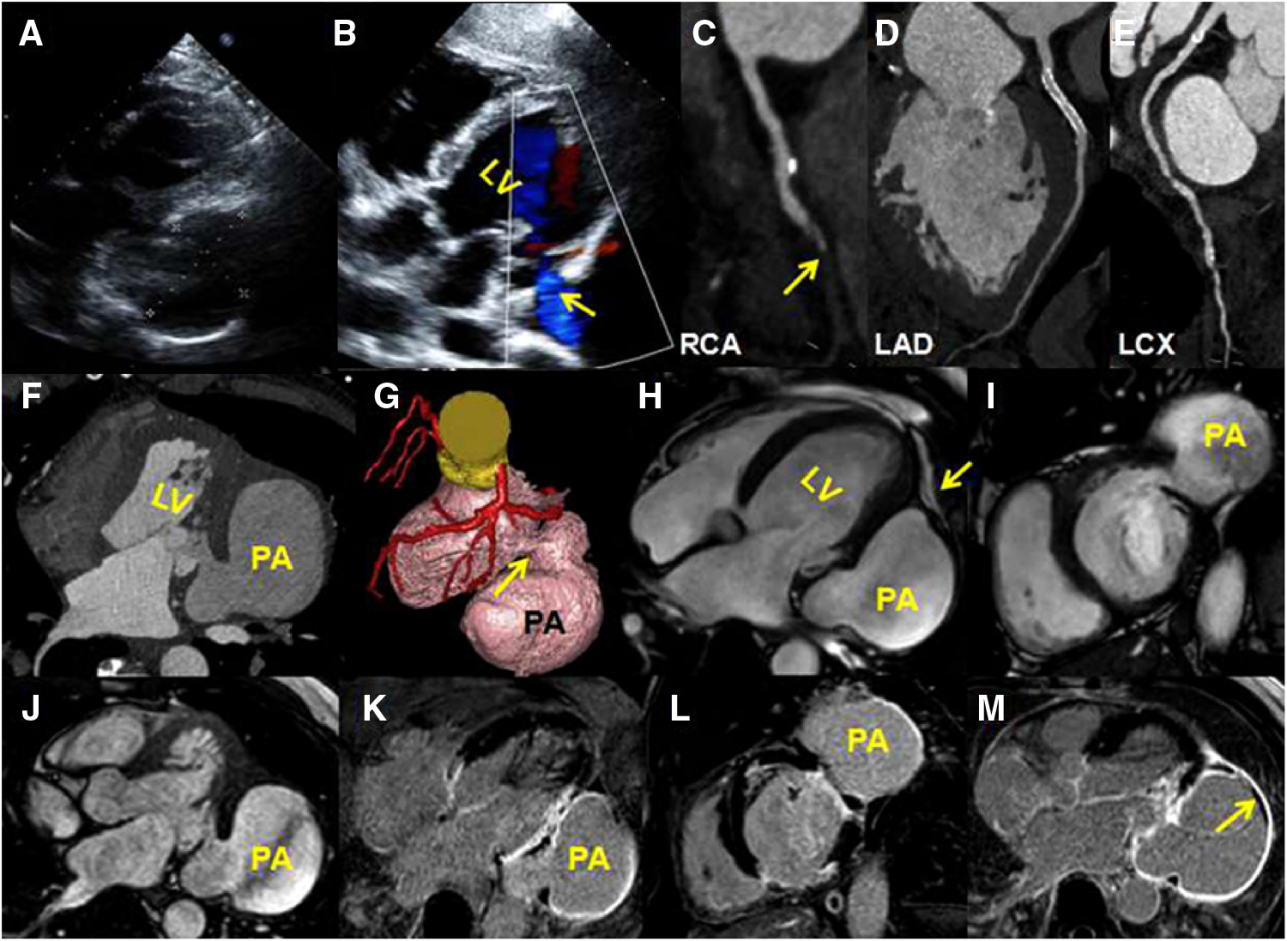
(A) A transthoracic four-chamber echocardiography image shows an aneurysm protruding from the posterior wall of the LV; the size of the aneurysm is 6.85 cm × 4.82 cm. (B) A color Doppler image shows a shunt from the left ventricle to the aneurysm (yellow arrow). (C–E) A coronary computed tomography angiography of curved planar reconstruction images show that the RCA mid-distal is in occlusion (C, yellow arrow), the LAD stent is unobstructed (D), and there is mild stenosis in the LCX mid-segment (E) (F) An axial CTA image shows that the lateral wall of the LV is interrupted continuously. (G) A volume-rendering CTA image shows a globular cavity and a narrow neck of the aneurysm (yellow arrow). (H–J) CMR four-chambered, short-axis and horizontal axis cine images show the aneurysm, and the wall of the aneurysm is continuous with the visceral pericardium (yellow arrow). (K–M) Late gadolinium enhancement images show a transmural delay enhancement of the lateral wall of the LV. The enhanced part of the ventricular aneurysm wall is composed of pericardial components. There is a small-sized thrombus around the ventricular pseudoaneurysm (M, yellow arrow).
